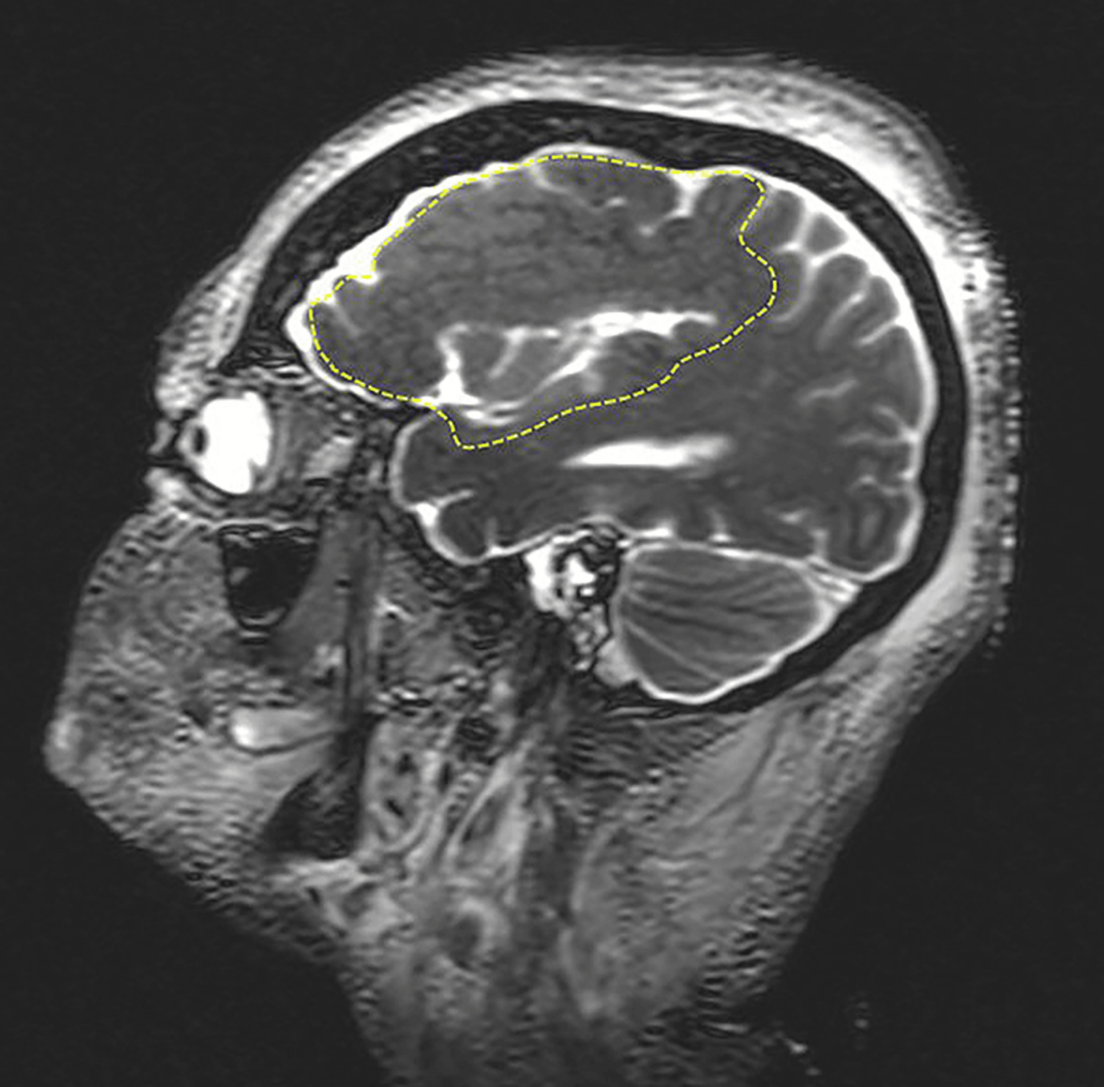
Magnetic resonance imaging of the donor head demonstrating the presence of polymicrogyria (PMG). Parasagittal image of a T2‐weighted MRI is shown. Note the aberrant boundary of the polymicrogyric cortex (depicted by stippled yellow lines) compared to the smooth gray‐white boundary in normal cortical areas.
